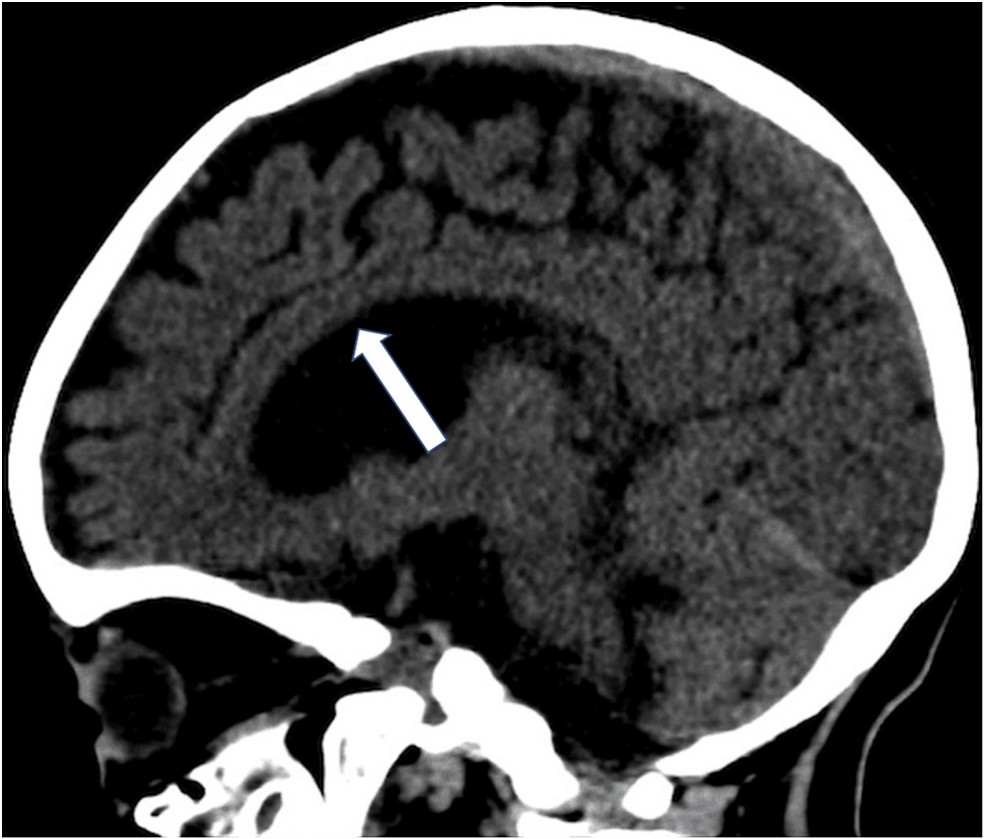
Non-enhanced CT brain sagittal section demonstrates corpus callosal thinning CT: computed tomography
